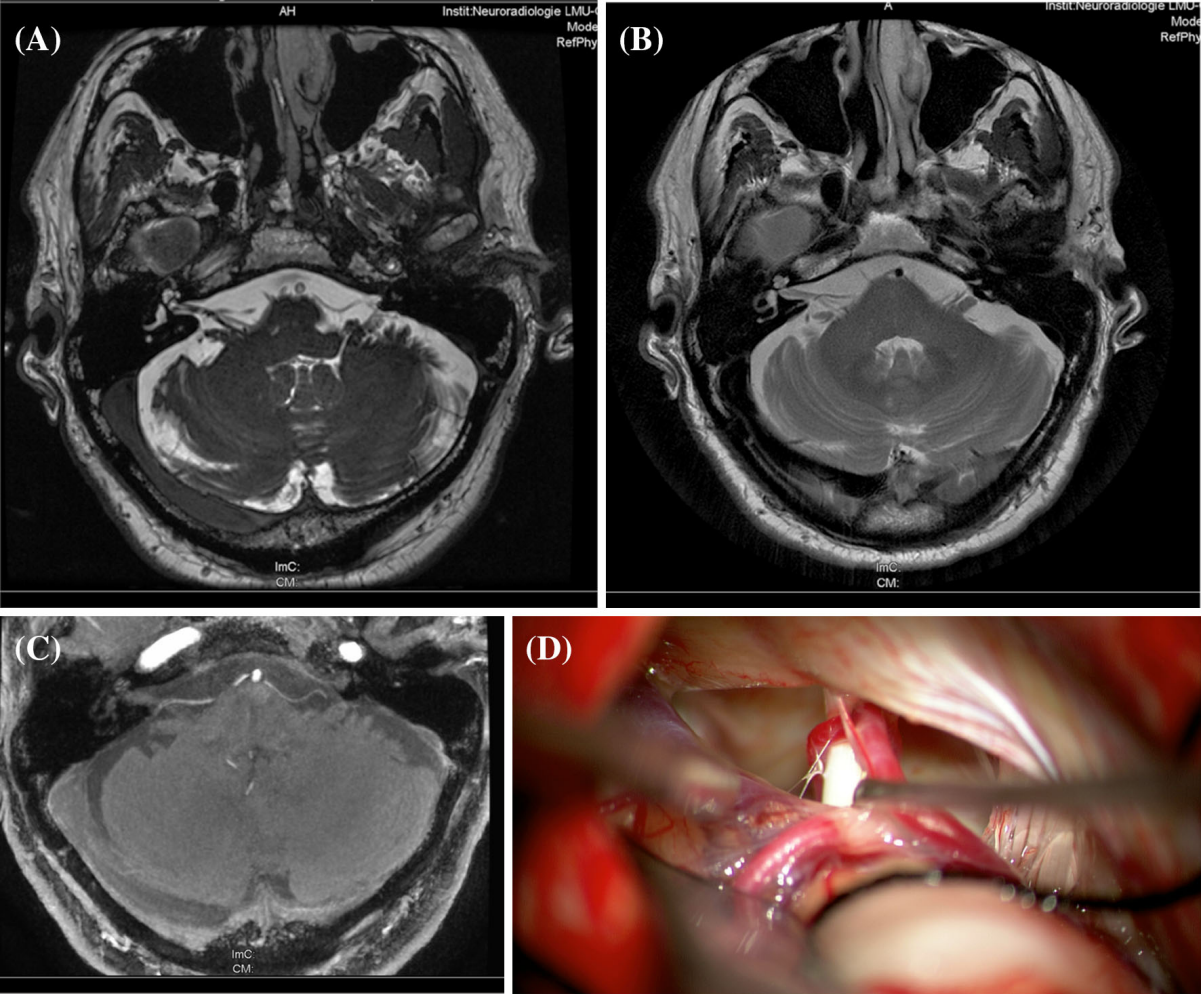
MRI of a patient with right-sided vestibular paroxysmia. a Fast imaging employing steady state acquisition sequence (FIESTA) and b T2 “propeller” and c time-of-flight MR-angiography demonstrate a neuro-vascular compression of the eighth cranial nerve by the AICA. This was also found intraoperatively (d) (modified from [23])
